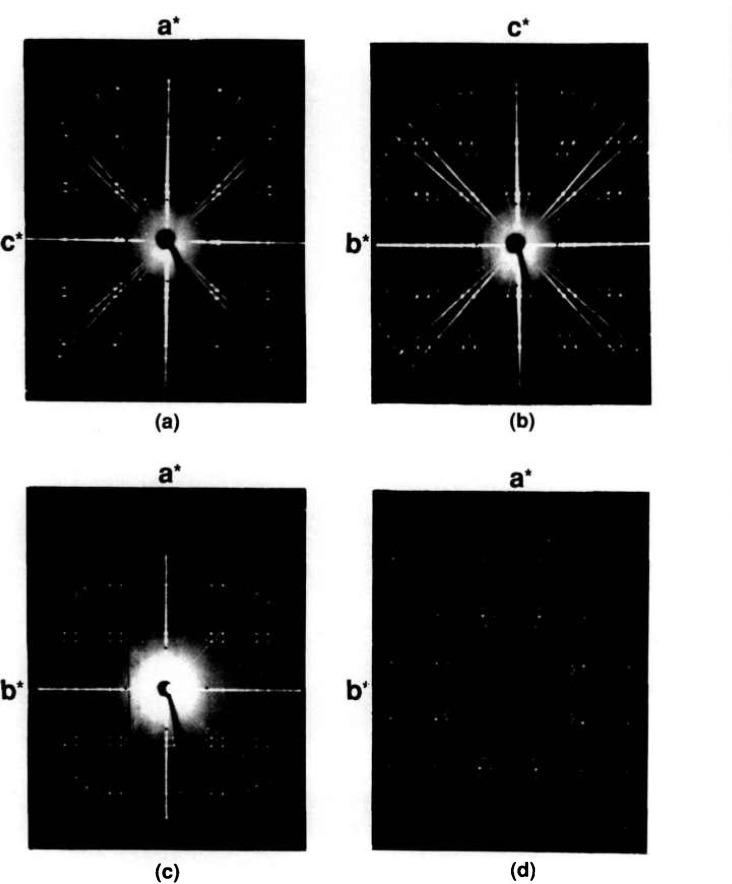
X-ray precession photographs of 8:4:5 (a) *h0l*, (b) *0kl*, (c) *hk0* and (d) *hkl.*
